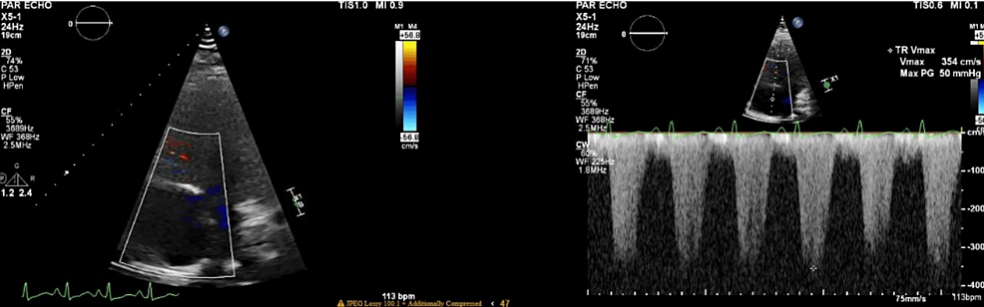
Transthoracic Echocardiogram Showing Tricuspid Regurgitant Max Jet Velocity (TR Vmax)
